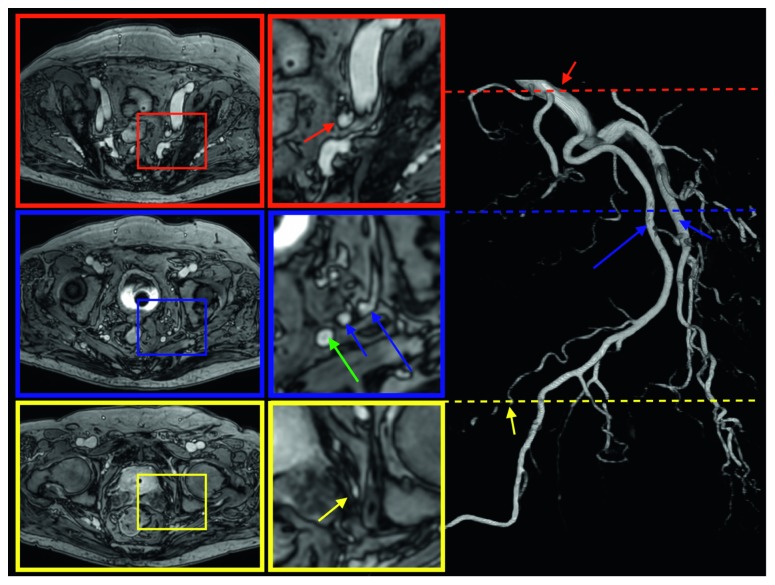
mDIXON postcontrast axial image of the prostate gland and corresponding angio-CT of arterial prostatic supply.
